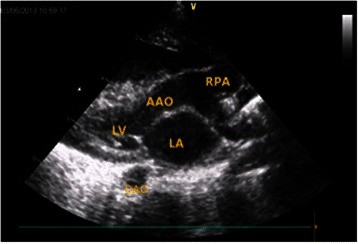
Preoperative echocardiograghy of Patient 3 showed that the right pulmonary artery arose from the ascending aorta. LA: left atrium, LV: left ventricle, AAO: ascending aorta, RPA: right pulmonary artery, DAO: descending aorta
